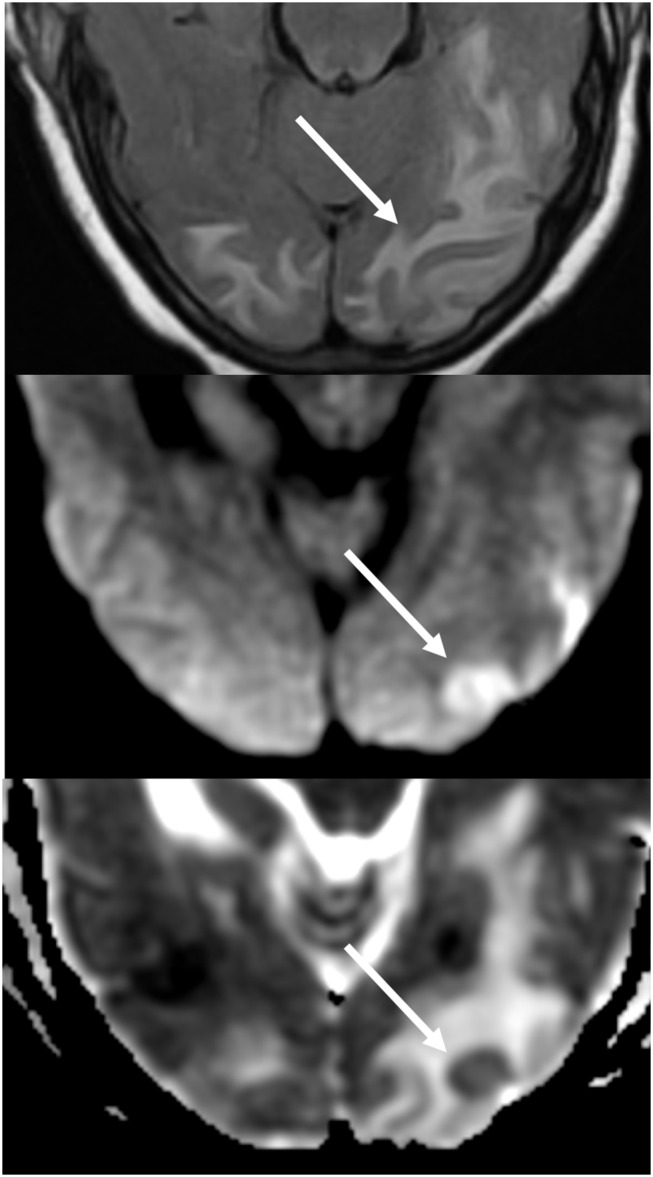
Patient with uncontrolled hypertension presenting with alteration of mental status. T2-FLAIR (top) image demonstrates edema in the occipital lobes. DWI (middle) and ADC map (bottom) images demonstrates a small arrow of restricted diffusion, with hyperintense signal on DWI and corresponding hypointensity on the ADC map.
